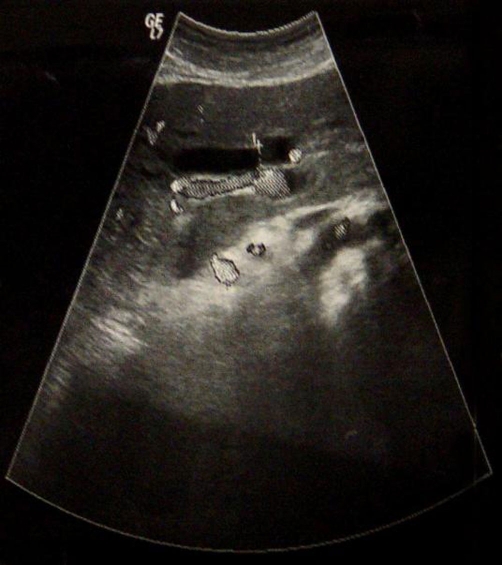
Abdominal sonography showed dilatation of the common bile duct.
